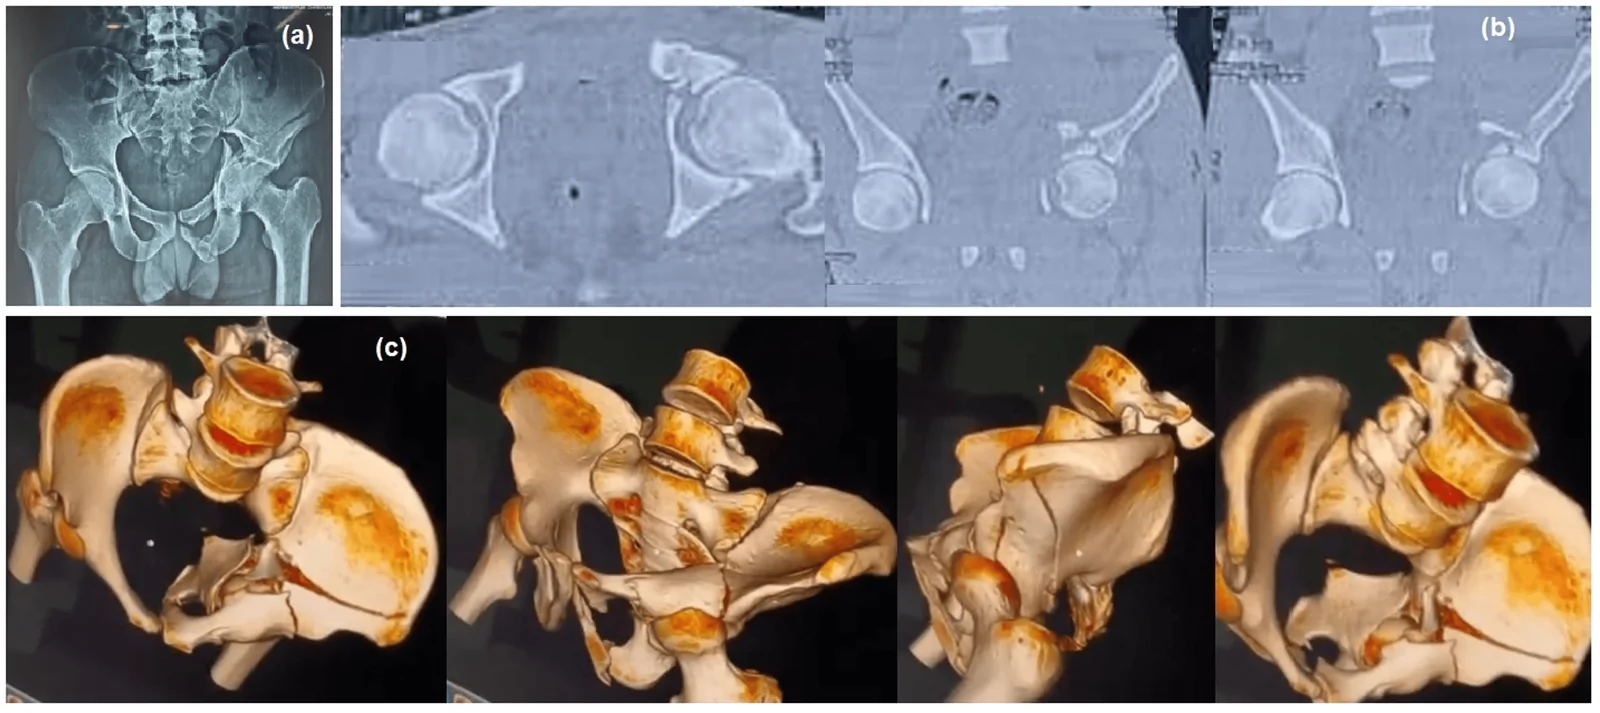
Case 1: Preoperative evaluation. (a) Anteroposterior pelvic radiograph demonstrating a central hip fracture-dislocation with a complex acetabular fracture. (b) Axial CT image showing medial displacement of the femoral head with involvement of the quadrilateral surface and anterior column. (c) Three-dimensional CT reconstruction demonstrating the complete fracture morphology, including fractures of the anterior column, quadrilateral surface, posterior column, and iliac blade.
